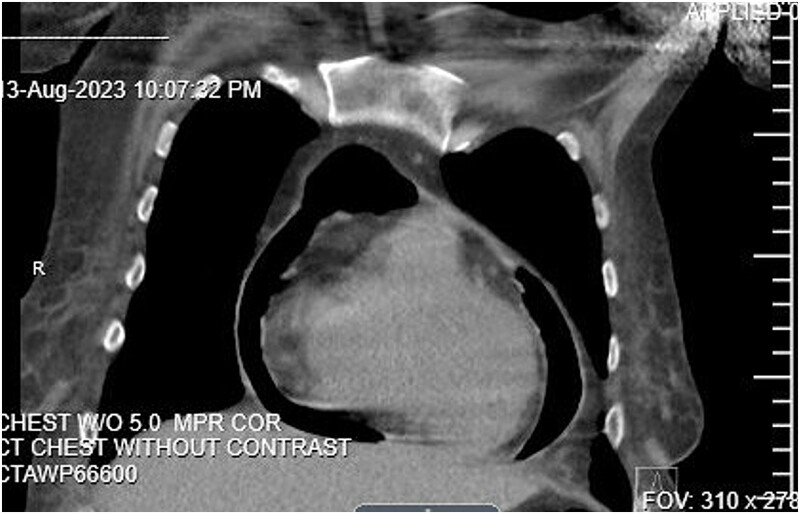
CT Abdomen pelvis showing moderate pneumopericardium observable from lower thorax.
